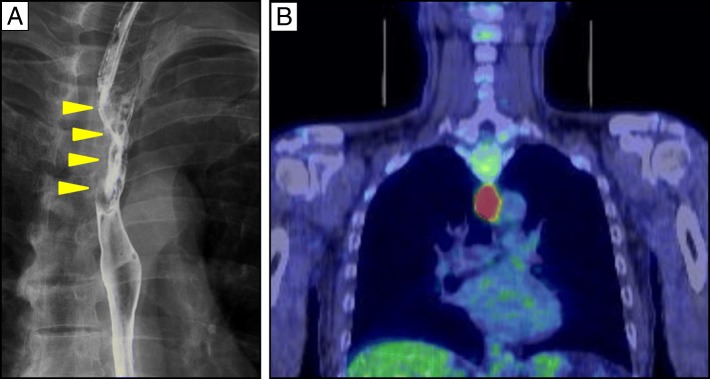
Preoperative images. a Esophagography showing the stenosed lesion in the upper thoracic esophagus (arrowheads). b PET-CT showing the accumulation of FDG at the lesion
